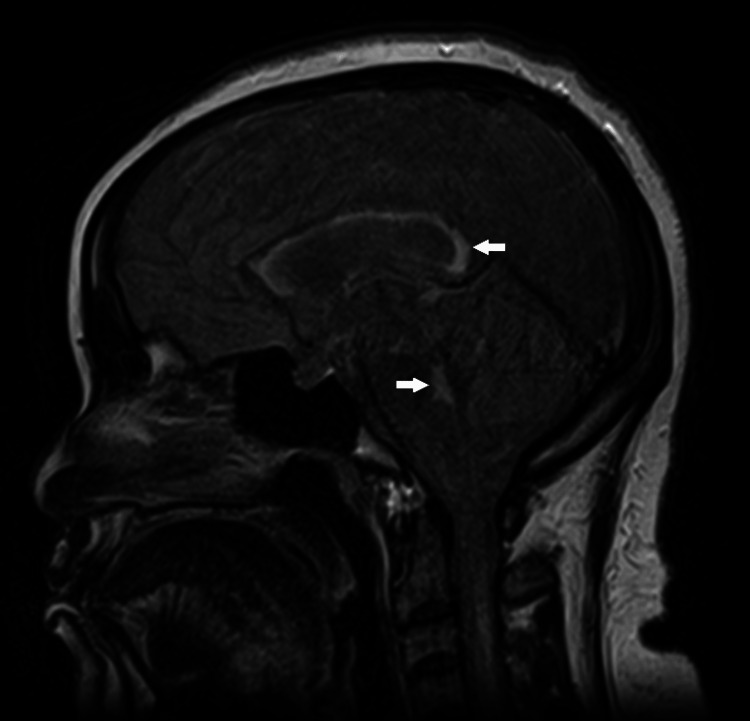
Sagittal MRI showing enhancements (white arrows) in the periventricular and dorsal pontine regions. MRI: magnetic resonance imaging
